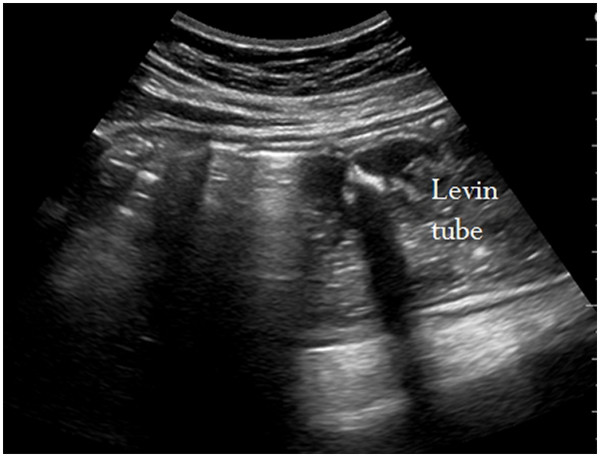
Sonographic visualization of a nasogastric tube. The weighted tip appears as a hyperechogenic line with a posterior acoustic shadow.
